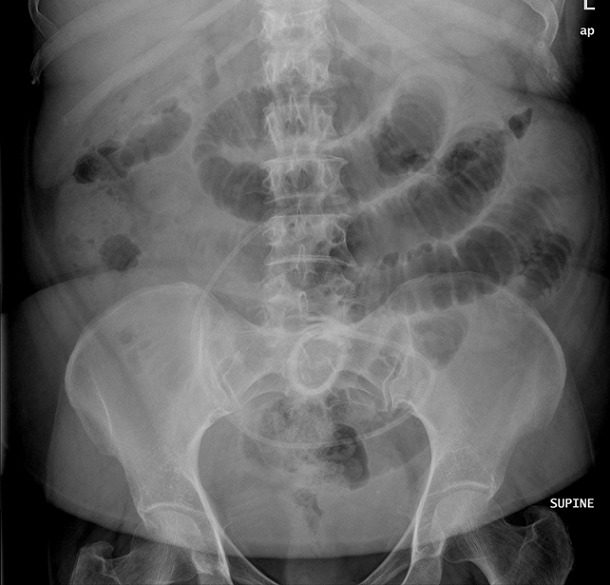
Supine abdomen X-Ray showed small bowel dilatation and ectopic lower abdominal position of gastric band.
